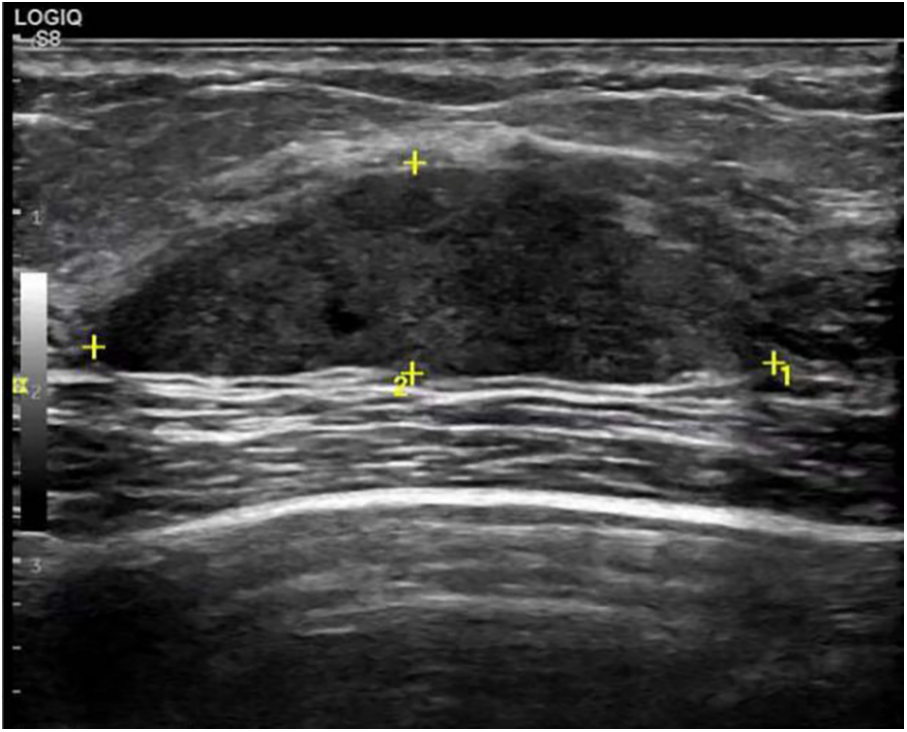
Color Doppler ultrasound showed a solid phyma, with regular shape and clear boundary.
